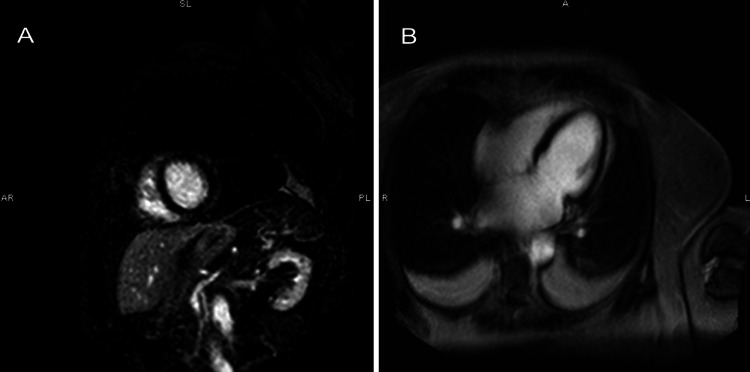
(A and B) LGE imaging MR images showing the absence of significant enhancement in the myocardium including the apical segment where evidence of inflammation was noted. LGE: Late gadolinium enhancement; MR: magnetic resonance
